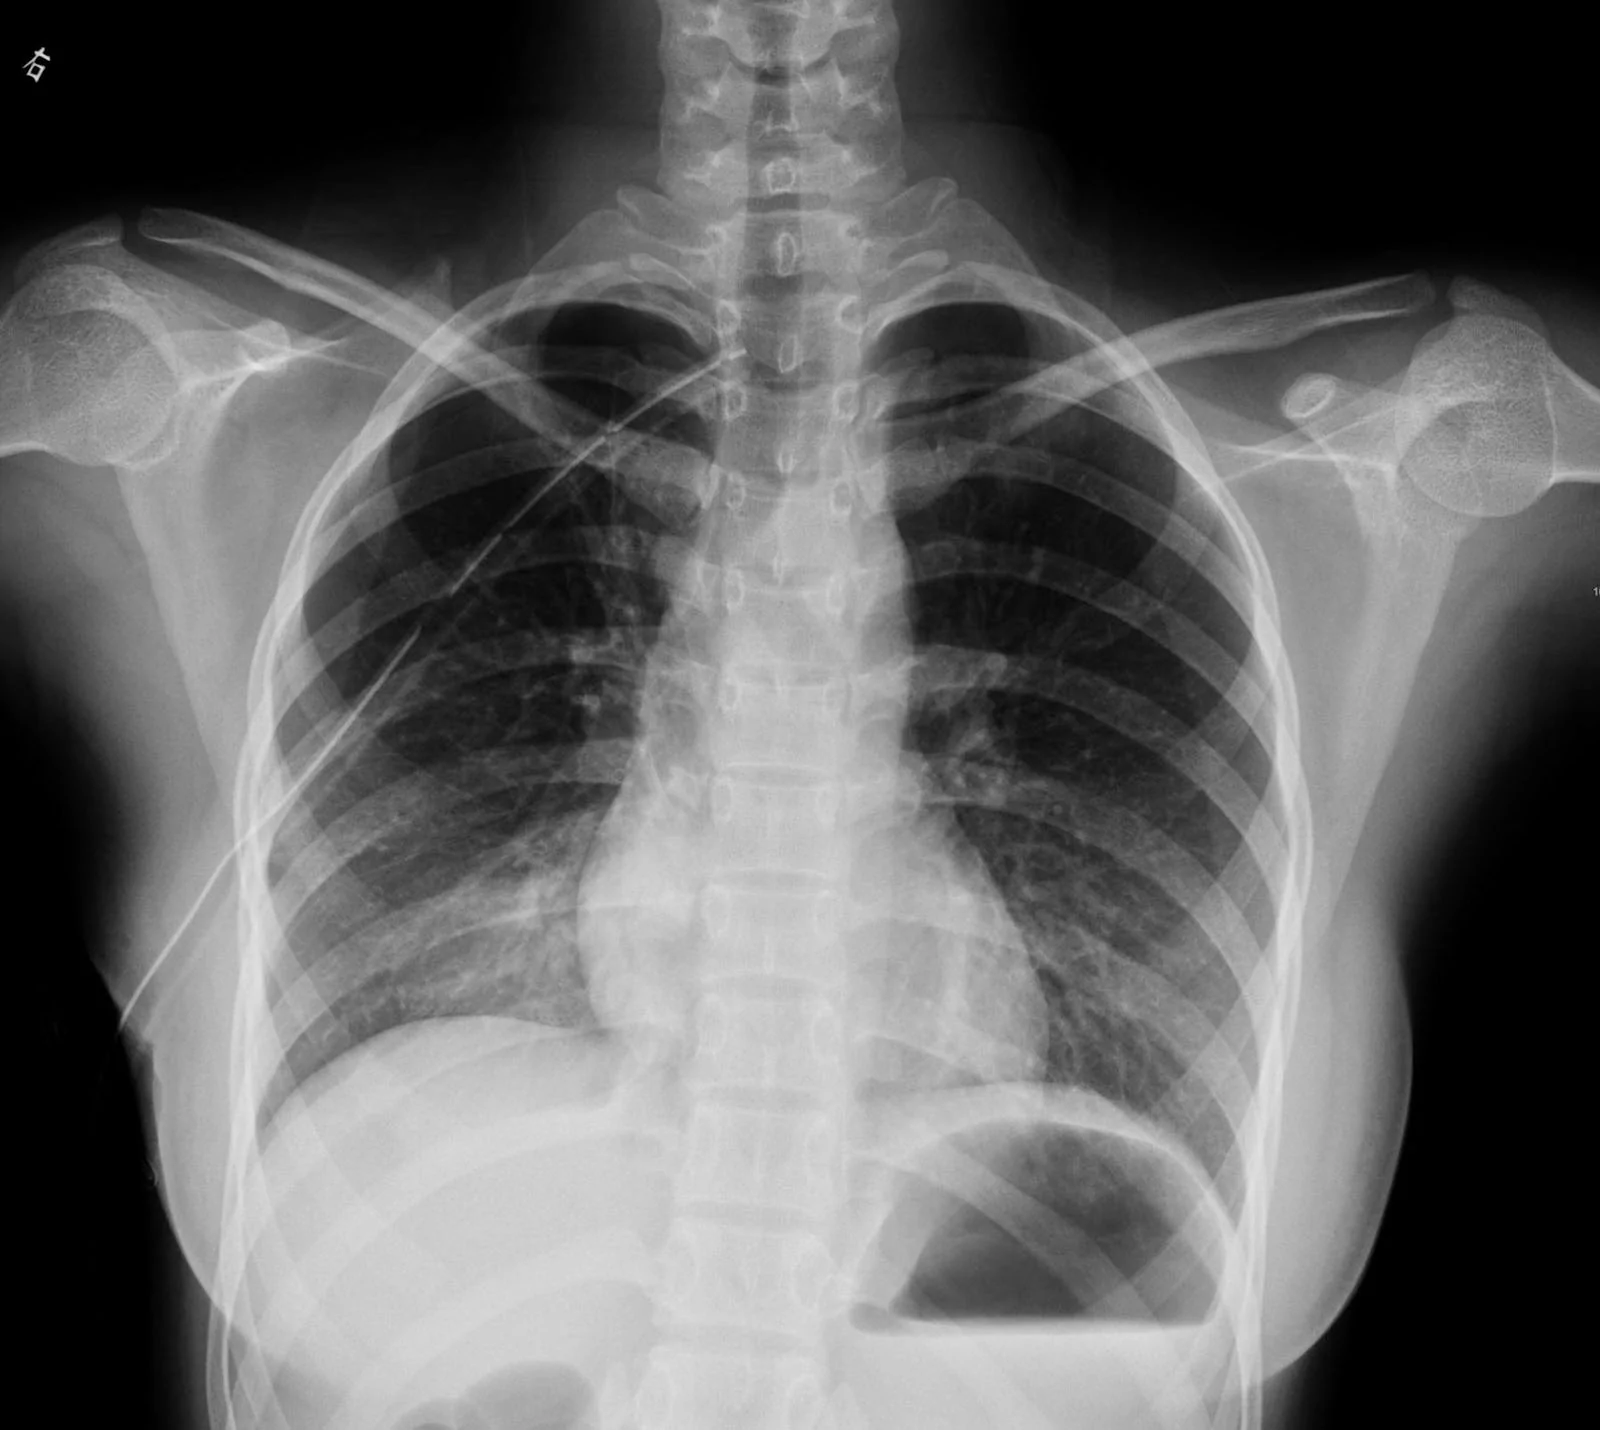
Postoperative day 1 chest X-ray demonstrates satisfactory lung re-expansion with no evidence of pneumothorax or pleural effusion. The foreign body shadow in the right lower lung has completely disappeared.
